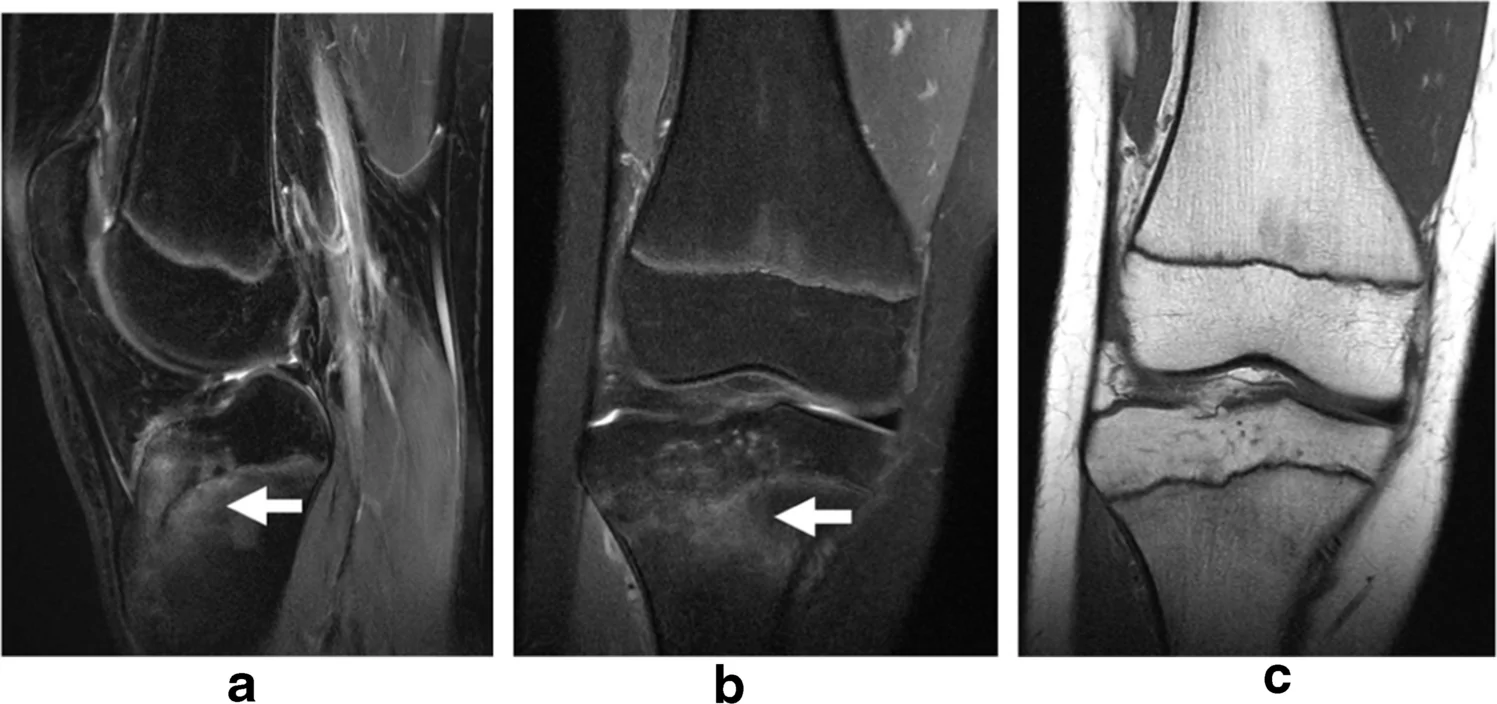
Imaged obtained in 17-year-old boy who presented with pain in the right knee following a contusion. a Sagittal T2 FS MR image shows mild hyperintense signal within the posterior aspect of the proximal tibial metaphysis consistent with mild bone marrow edema (arrow). b Coronal PD FS MR image of the left knee shows patchy areas of hyperintense signal in the metaphyseal region of the proximal tibia (arrow). c Coronal T1MR image shows normal T1 marrow signal with intact cortex and preserved cartilage of the proximal tibia
